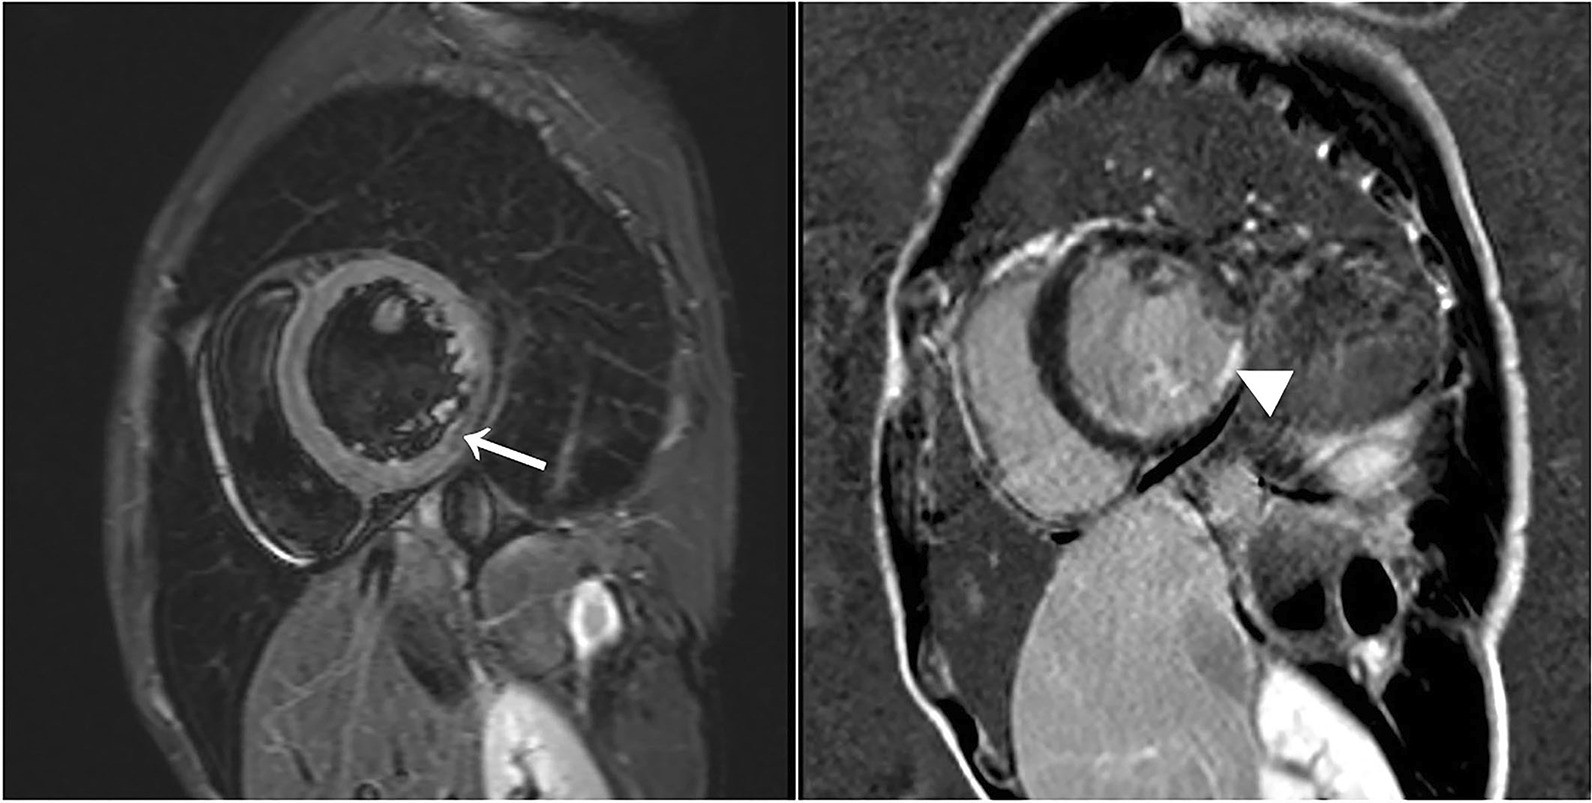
Hypointense on dark blood T2-weighted image (arrow) and late gadolinium enhancement (arrow head) on cardiac magnetic resonance imaging
